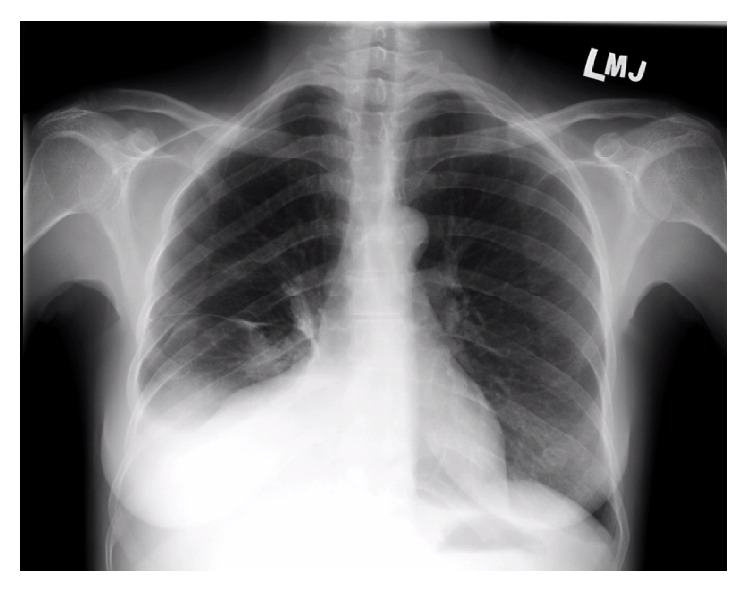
Chest X-ray showing improvement of pleural effusions after abdominal paracentesis, suggesting that the fluid was communicating between pleural and peritoneal cavities.
